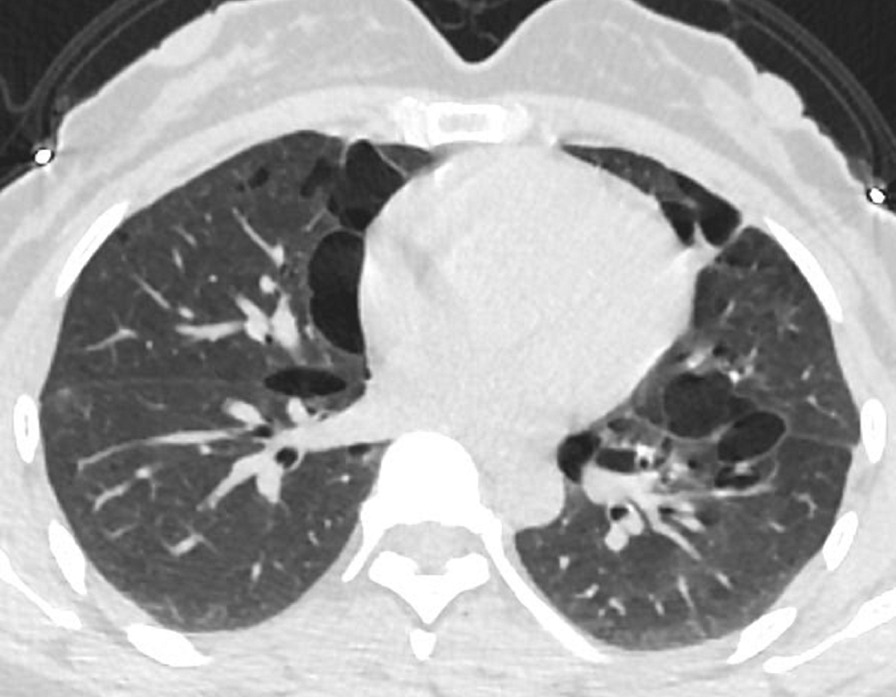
Chest CT images of patients diagnosed with BHD syndrome. Multiple well-circumscribed, thin-walled lung cysts of willow-like, oval, and irregular shapes and varying sizes can be seen. The lung cysts are in the mediastinal subpleural and interlobular fissure areas in both lungs and grow near the mediastinum. Figures 1 and 2 are images of the same patient before (Fig. 1) and during (Fig. 2) pneumothorax
